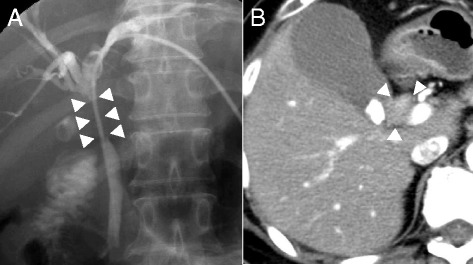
Preoperative imaging of hilar cholangiocarcinoma. a Stenosis of proximal biliary tract is appreciated through percutaneous transhepatic biliary drainage catheter. b Computed tomography shows the tumor with slight enhancement. *Arrow* represents the tumor
